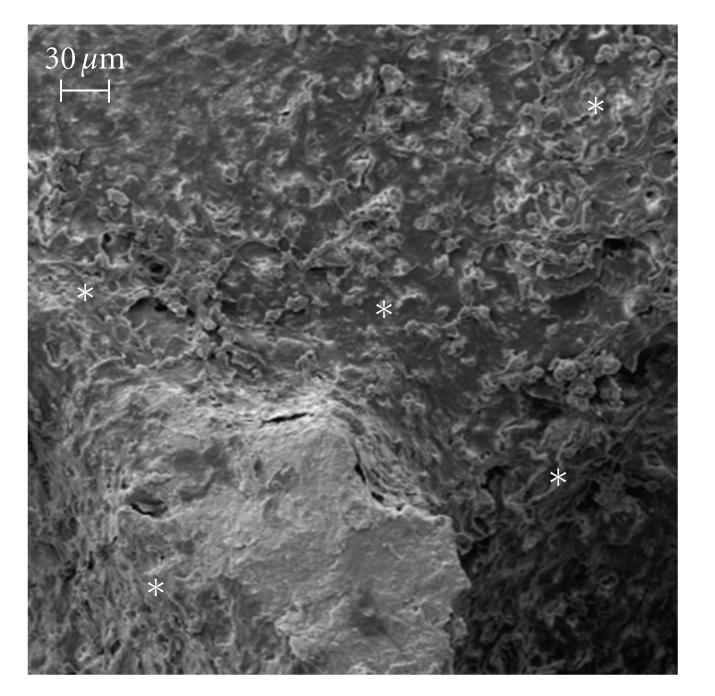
SEM image of the ultrasonic culture, bar equal to 30 *μ*m. During the culture period, the physical stimulus caused a wide-ranging coat of the internal surface of the biomaterial: several osteoblasts proliferated and the biomaterial was tending to be hidden by cell-matrix layers (asterisks).
